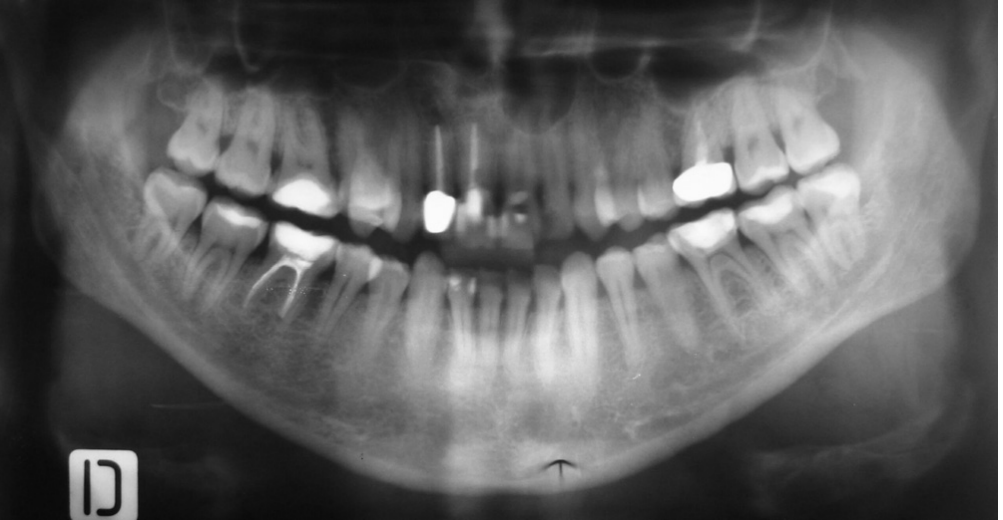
Panoramic tomography revealed the periapical lesion associated with teeth 21,22.
